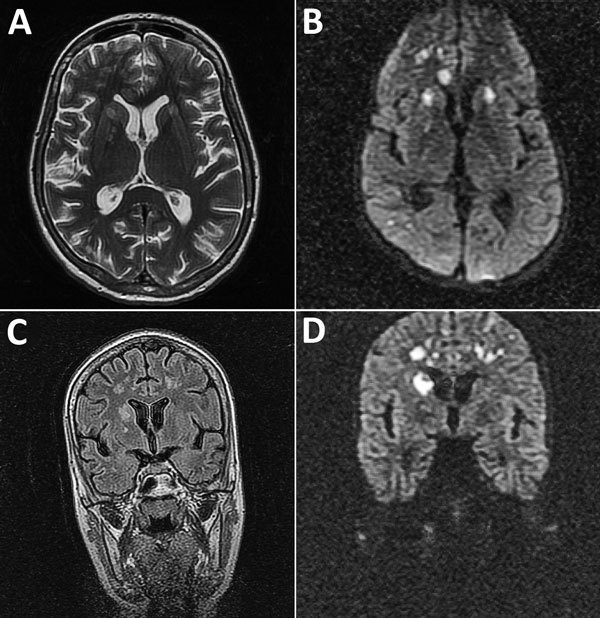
Magnetic resonance imaging of the brain of a 28-year-old woman (patient 1) who had neurologic complications of influenza B virus infection, Romania. A) Axial T2 image showing multiple areas of T2-associated hyperintense lesions with involvement of the genu corpus callosum, bilateral internal capsule, and several areas of white matter in the right frontal lobe, and more discreetly at the limit between the right parietal and occipital lobe. B) Axial diffusion-weighted image showing restricted diffusion associated with lesions. C) Coronal fluid–attenuated inversion recovery image showing multiple hyperintense lesions in the right caudate head and the cortical and deep white matter of the frontal lobes. D) Coronal diffusion-weighted image showing restricted diffusion associated with lesions.
